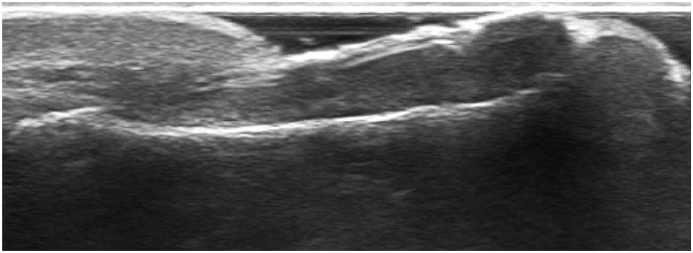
Ultrasound imaging of a bone spur of a distal phalanx with a nail growth problem.
